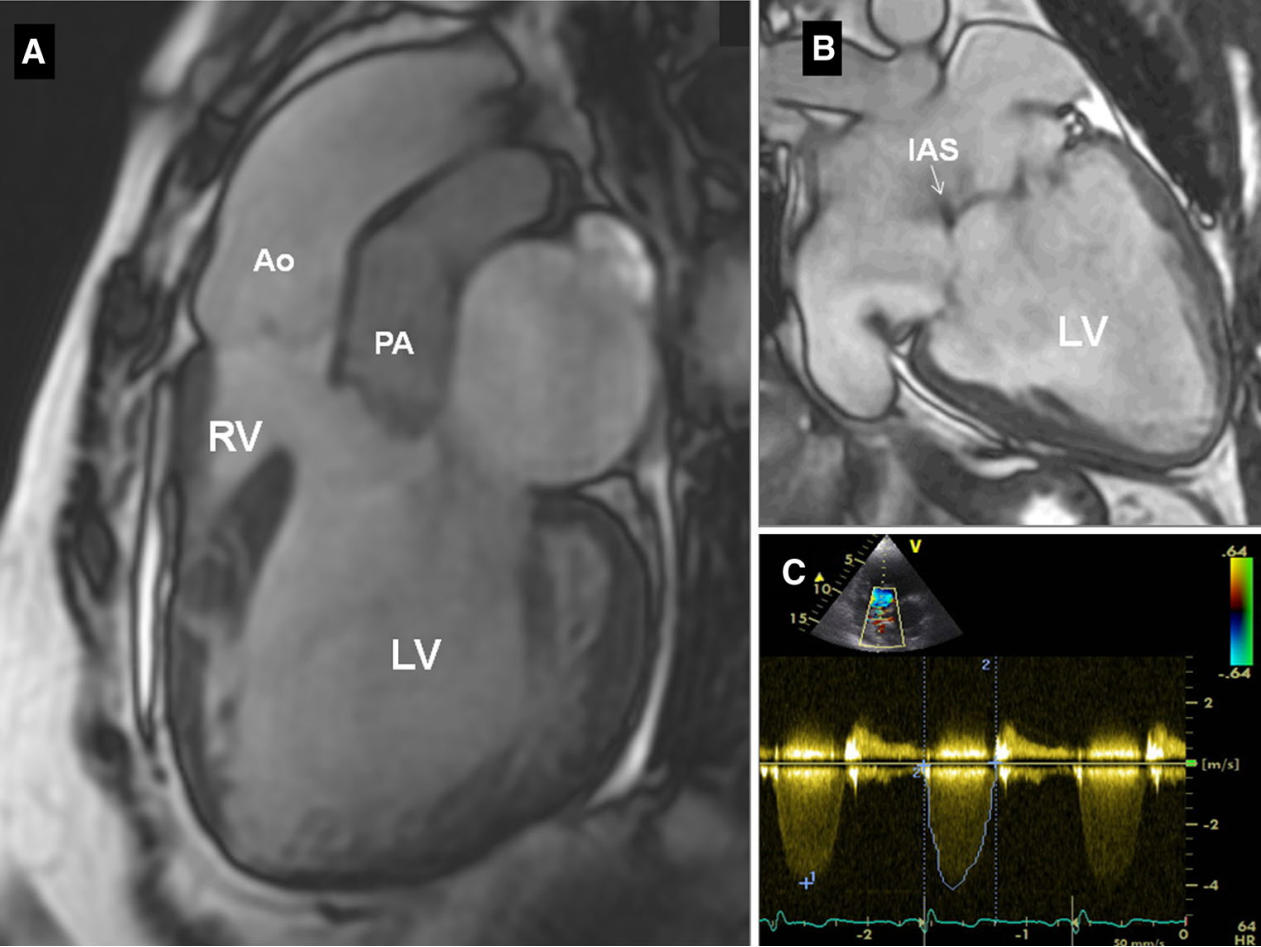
Cardiac magnetic resonance (a, b) and transthoracic echocardiography (c) showing a left-type single ventricle (*LV*), an unrestrictive interventricular septum defect with a rudimentary right ventricle (*RV*), transposition of great arteries (*Ao* aorta;* PA* pulmonary artery), residual interatrial septum (*IAS*) (b) and a moderate-to-severe pulmonary stenosis in pulsed wave Doppler (c)
